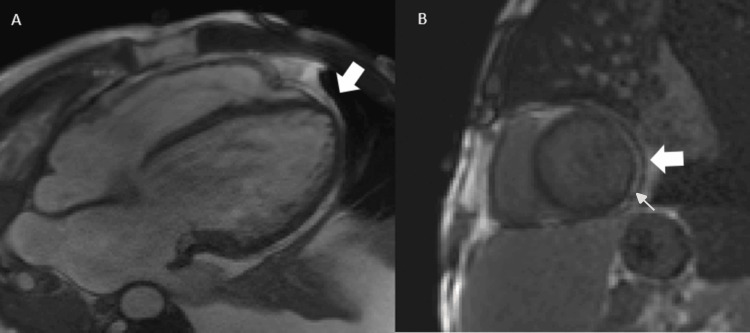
Cardiac magnetic resonance imaging (CMR) (A) Four-chamber cine sequences demonstrated a global increase in pericardial thickness (white arrow), measuring up to 6 mm in the region surrounding the left ventricle. (B) Short-axis late gadolinium enhancement (LGE) sequence showing pericardial enhancement (large white arrow) and lateral subepicardial left ventricular (LV) enhancement consistent with myocardial involvement (small white arrow). These findings are consistent with myopericarditis.
